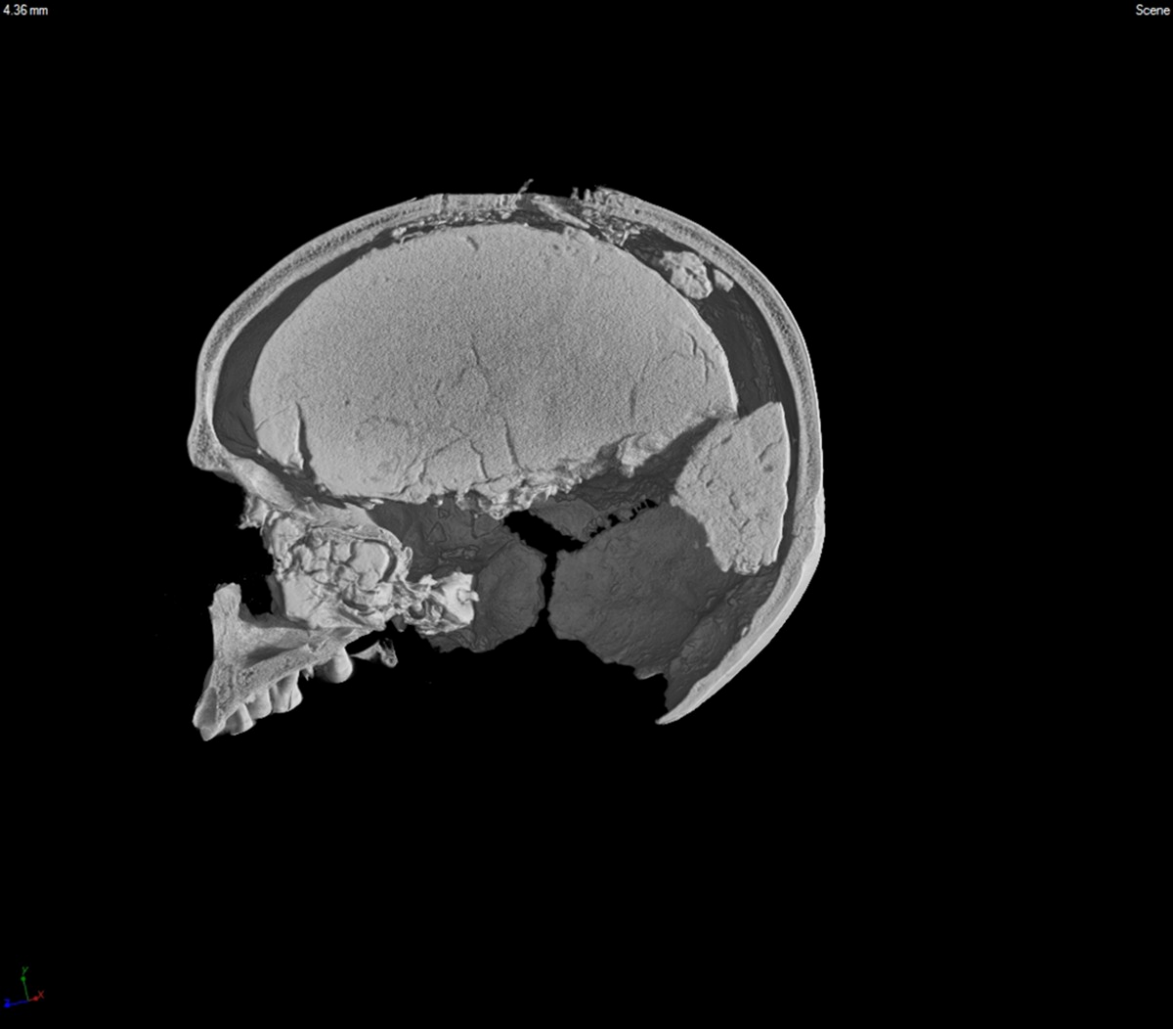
MLC—CT scan with sediment filling the endocranial cavity.
